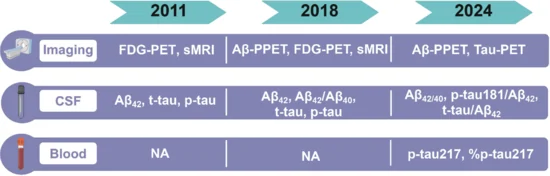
Diagnostic criteria for AD according to NIA-AA. The NIA-AA published diagnostic criteria for AD that include imaging, cerebrospinal fluid (CSF), and blood-based biomarkers (BBMs) used for diagnosis. These criteria were updated in 2011, 2018, and 2024, respectively. The imaging and CSF biomarkers utilized for diagnosis have evolved over time. Notably, BBMs were included in the updated 2024 criteria for diagnosing AD. Created in BioRender. Cui, Z. (2025) https://BioRender.com/put1olm. AD: Alzheimer’s disease; Aβ: amyloid-β; Aβ-PET: Aβ positron emission tomography; BBMs: blood-based biomarkers; CSF: cerebrospinal fluid; FDG-PET: fluorodeoxyglucose positron emission tomography; GFAP: glial fibrillary acidic protein; NA: not available; NfL: neurofilament light chain; NIA-AA: National Institute on Aging Alzheimer’s Association; p-tau: phosphorylated tau; sMRI: structural magnetic resonance imaging; tau-PET: tau-protein positron emission tomography.
